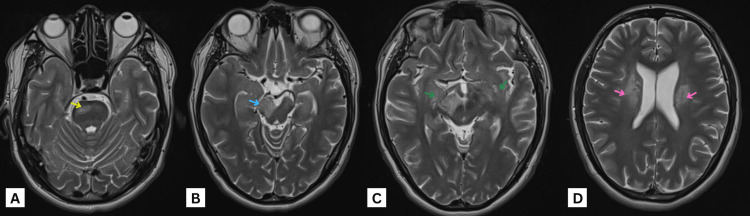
MRI of the brain axial T2 images at the level of the (A) pons, (B) midbrain, (C) upper midbrain, and (D) corona radiata, showing bilateral, almost symmetrical, signal alteration in the form of T2-FLAIR high-signal intensity involving the globus pallidus, corticospinal tracts (green arrows), and corona radiata (pink arrows). It starts from the corona radiata superiorly at the level of lateral ventricles extending to cerebral peduncles of the midbrain (blue arrows) inferiorly, affecting the right side more at this level. Similar signal alteration is also noted at the right anterolateral side of the pons (yellow arrows). No diffusion restriction can be seen. MRI: magnetic resonance imaging, FLAIR: fluid-attenuated inversion recovery
